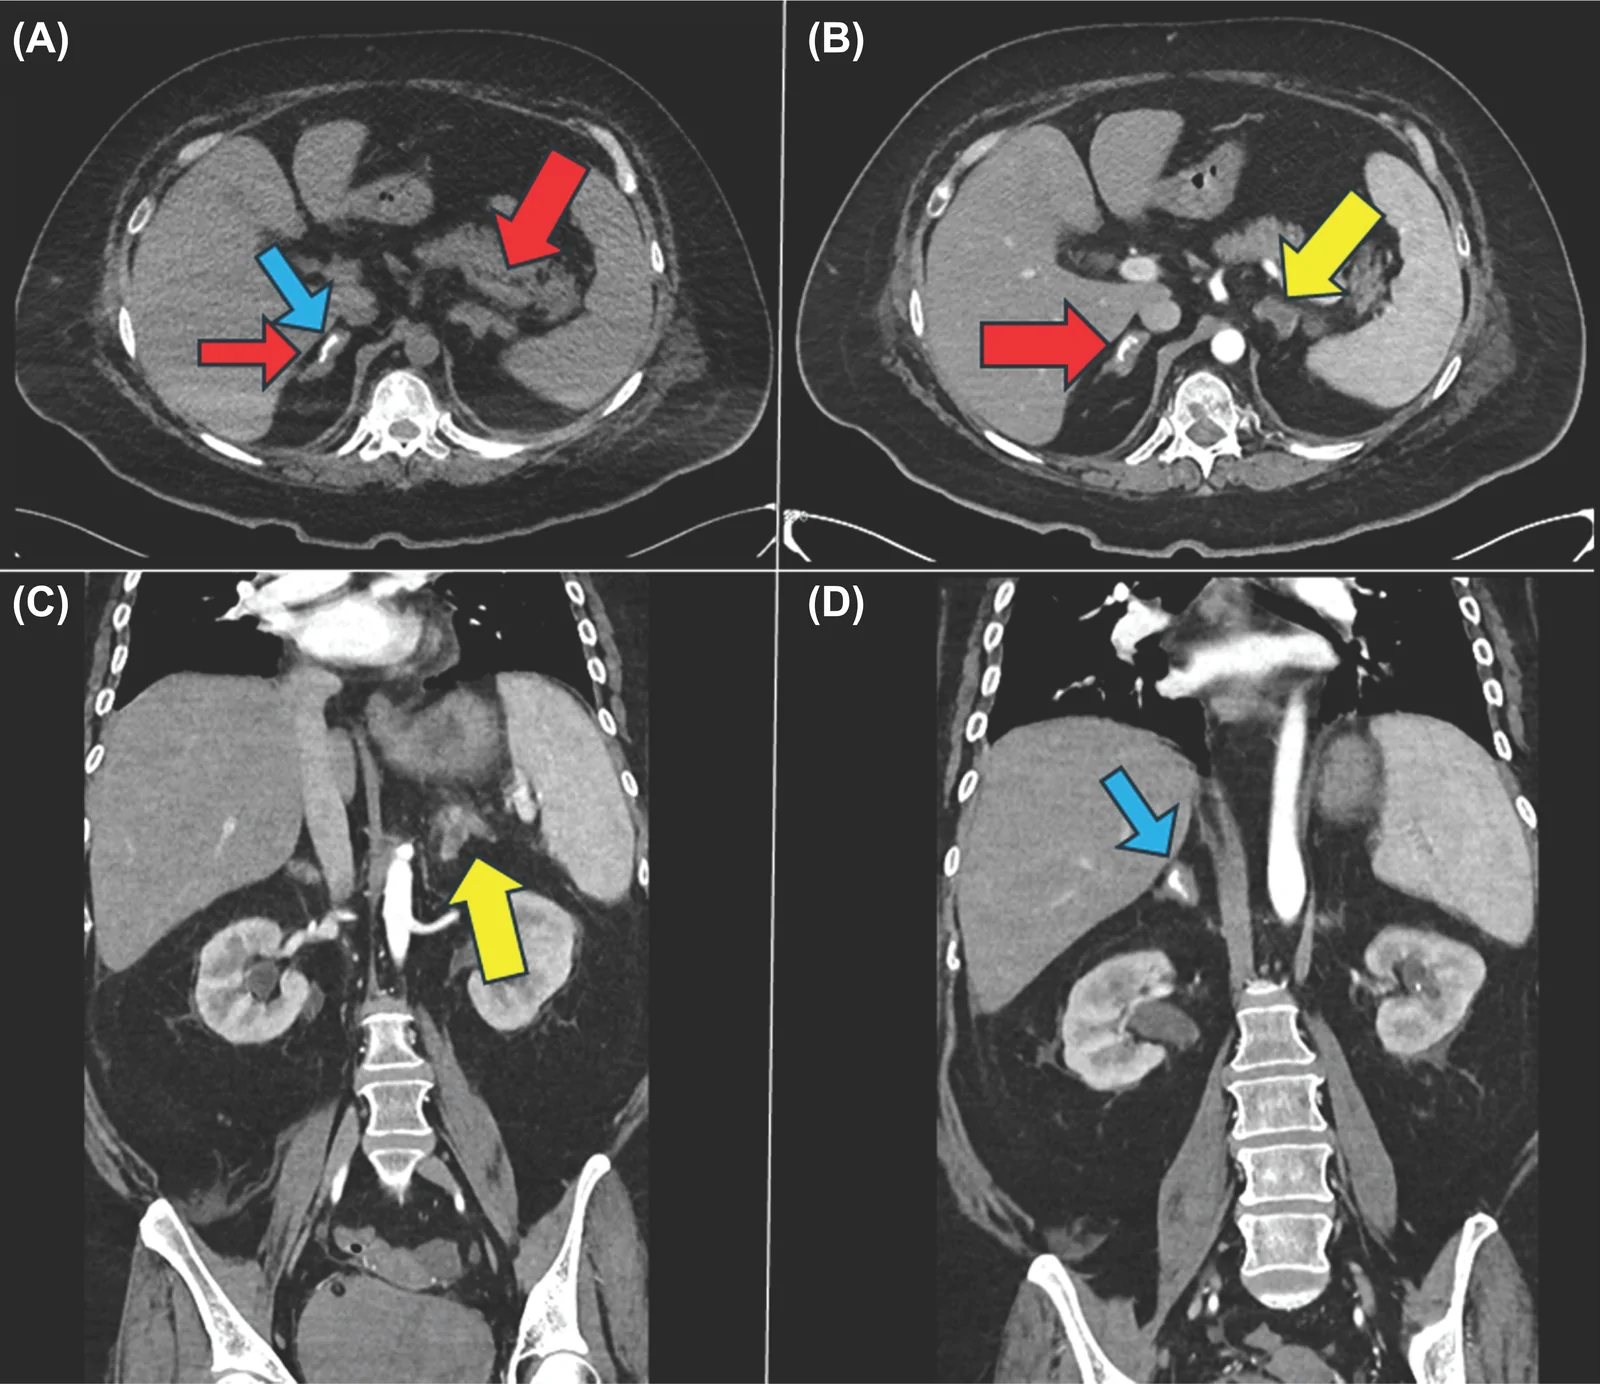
Abdominal CT showing non-contrast axial (A), contrast-enhanced axial (B), and coronal reconstructions (C, D). Bilateral adrenal hypertrophy (red arrows) with right-sided calcifications (blue arrow) and areas of reduced contrast enhancement/hypoperfusion (yellow arrows). Abbreviation: CT, computed tomography.
